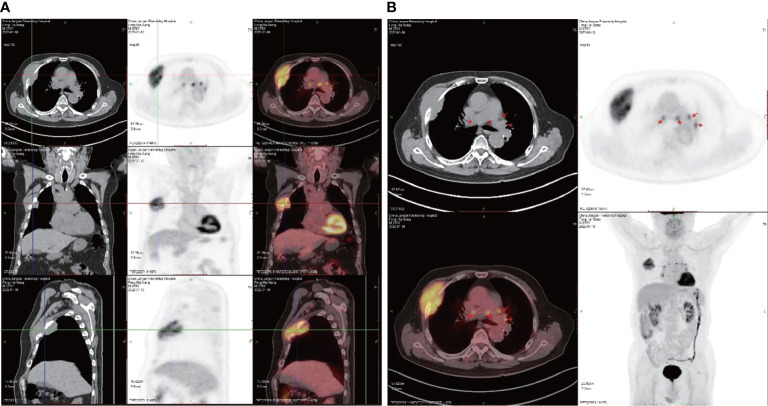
Positron emission tomography (PET) CT scans. (A) The tumor in frontal, lateral, and transverse section. (B) Multiple lymph nodes showing fluorodeoxyglucose uptake in the mediastinum and hilum (SUVmax 7.4).
